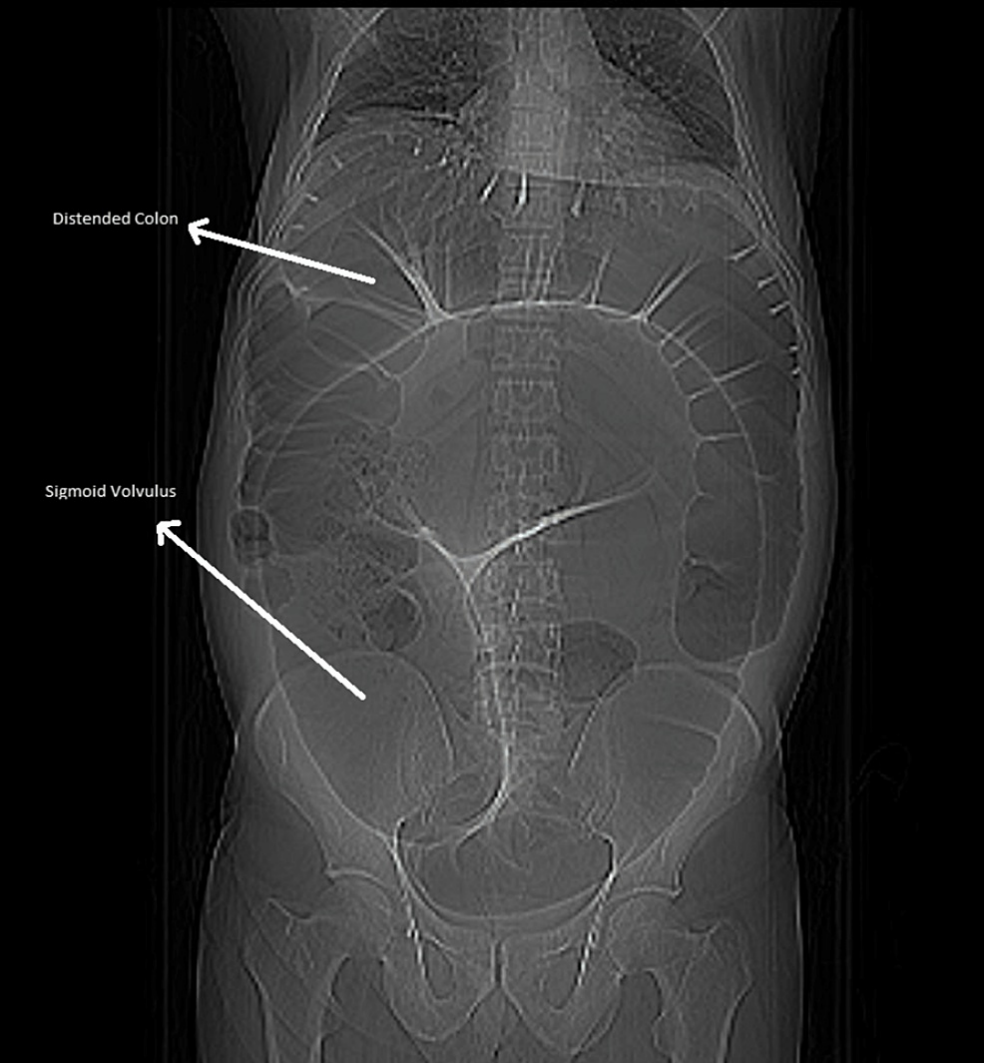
X-ray scan showing a grossly distended loop of the colon with sigmoid volvulus with air under the right side of the diaphragm
